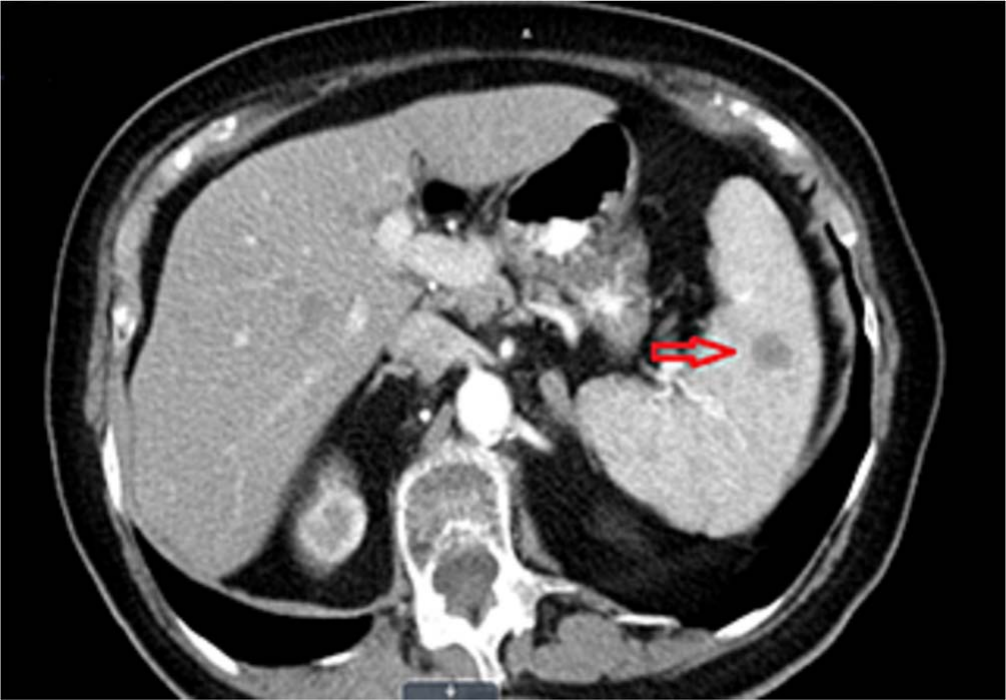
Transverse CT scan plane depicting hypodense splenic lesion (arrow).
